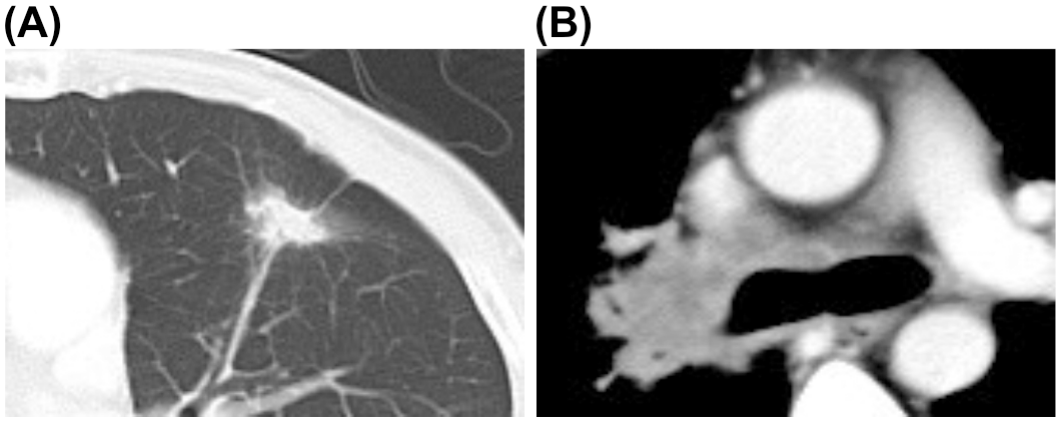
Computed tomography images of a lung adenocarcinoma showing ground-glass opacity with solid components. (A) Thoracic computed tomography reveals ground-glass opacity with solid components. (B) Thoracic computed tomography reveals extranodal invasion of the lymph nodes.
